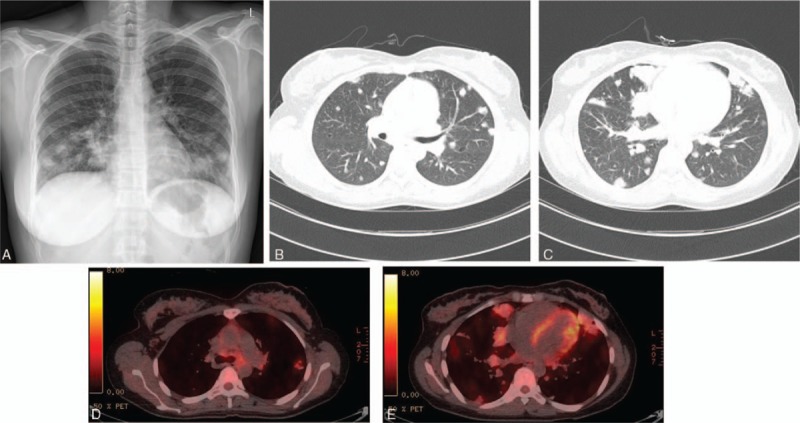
(A) Chest x-ray revealed multiple ill-defined nodules in both lung fields. (B, C) Chest CT revealed multiple randomly distributed lung nodules in both lung fields. (D, E) PET-CT revealed increased FDG uptake in multiple both lung nodules. CT = computed tomography, FDG = fluorodeoxyglucose, PET = positron emission tomography.
